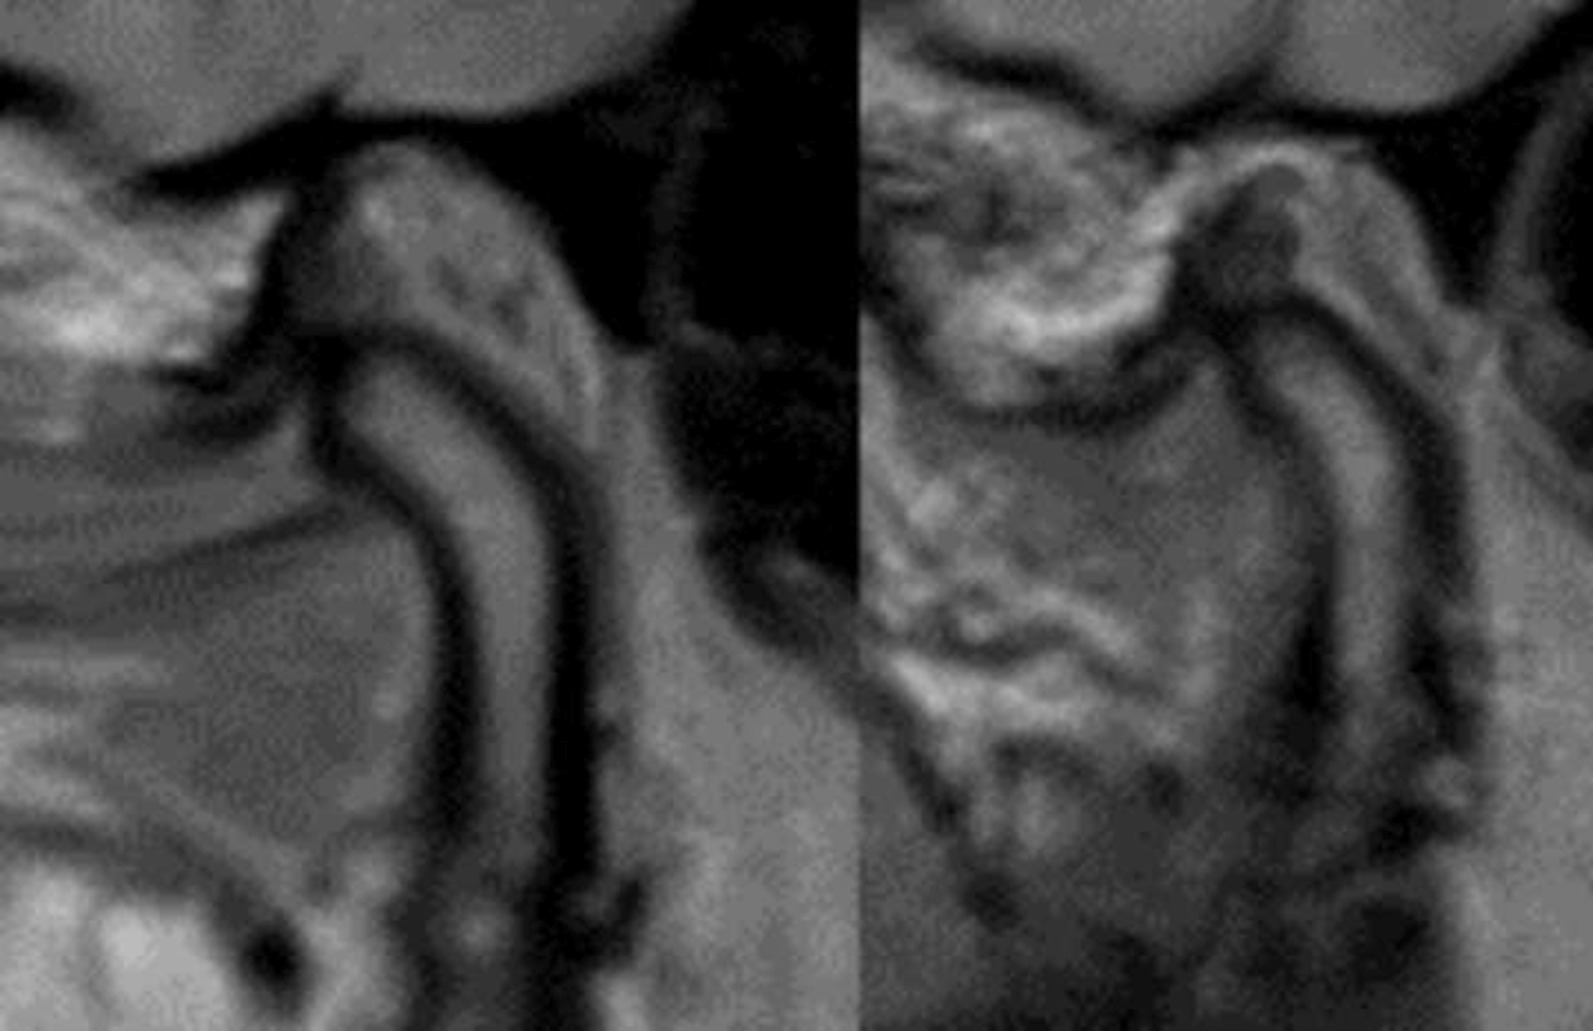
MRI open mouth left (12/19)
